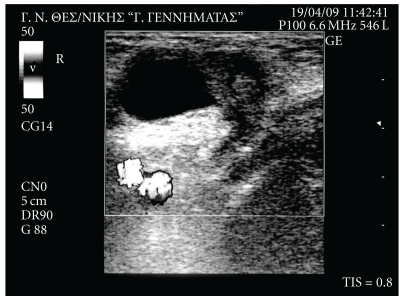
Ultrasonography showing the oedematous hernia sac, above the femoral vessels.
